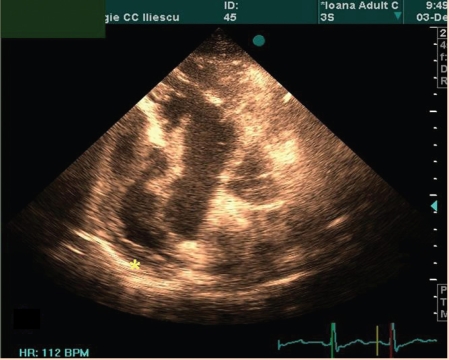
Apical four–chamber recording of a large right atrium and enlarged right ventricular cavity. Diastole before the atrial depolarization. The long masses arise in the posterior and lateral right atrial wall. (asterix)  The tumor extends into the atrial cavity and through the opened tricuspid valve, into the right ventricular inflow.
